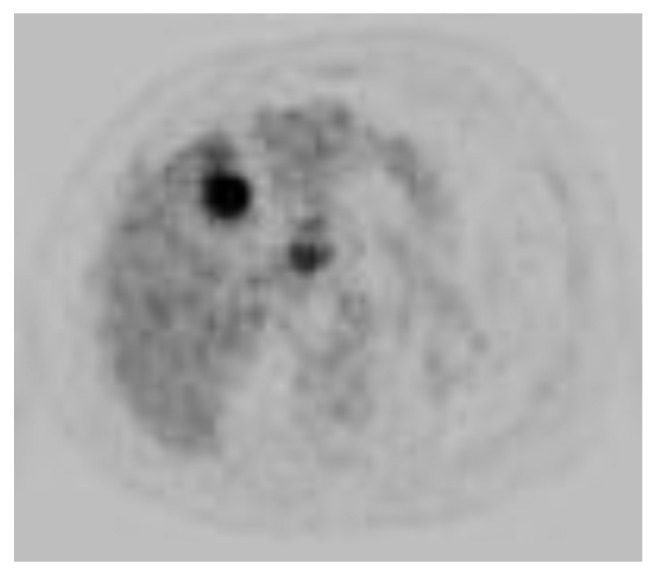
PET-CT demonstrated 3 cm hypermetabolic liver mass seen on MRI.
